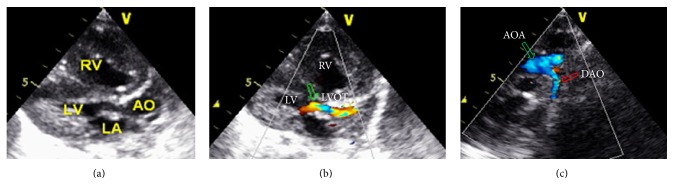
Infant echocardiogram images at 125 days. Echocardiogram in a left ventricular long axis view, demonstrating severe left ventricular outflow tract (LVOT) obstruction and biventricular hypertrophy (a). Doppler color flow image, displaying turbulence flow through LVOT (b, green arrow). Doppler color flow image in the suprasternal long axis view of the aortic arch, demonstrating low velocity laminar flow through the coarctation of the DAO (c, red arrow). AO: aorta; LA: left atrium; LV: left ventricle; RV: right ventricle; LVOT: left ventricular outflow tract; AOA: aortic arch; and DAO: descending aortic.
